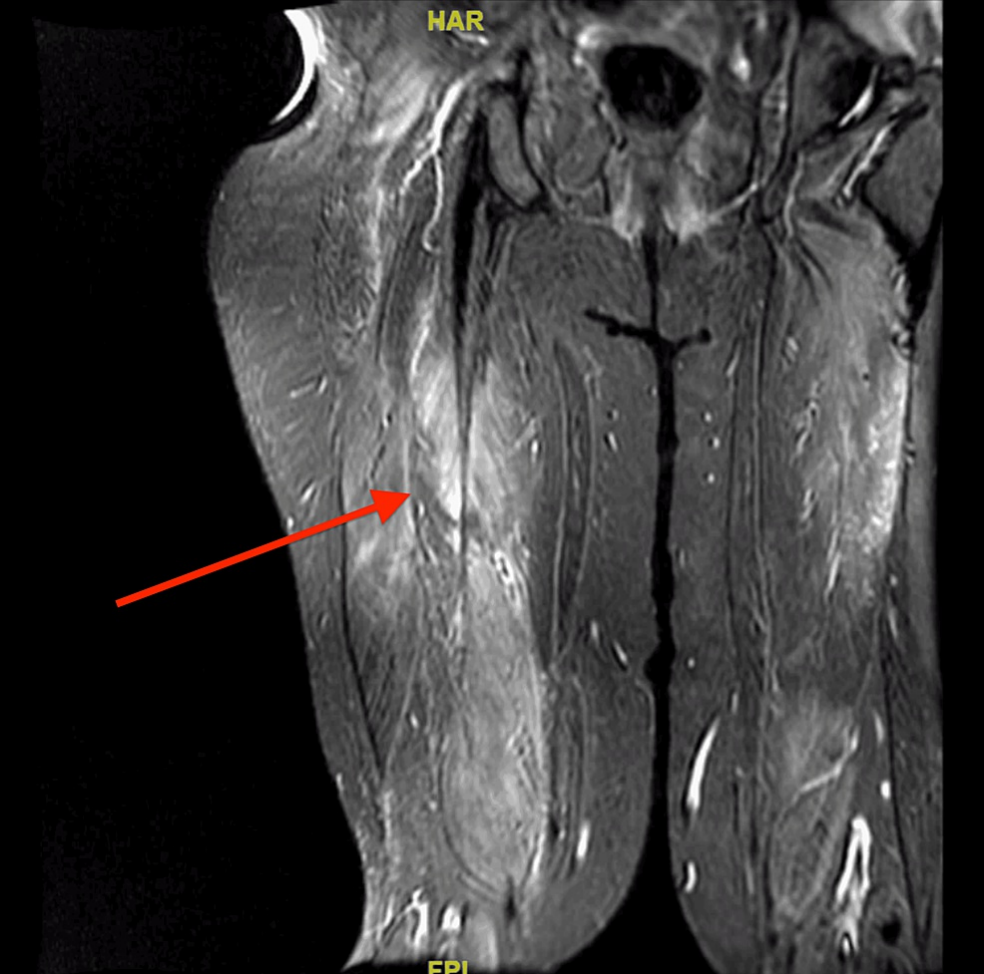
MRI of bilateral lower extremities showing fairly symmetric moderate bilateral myositis throughout bilateral thigh The red arrow shows myositis.
